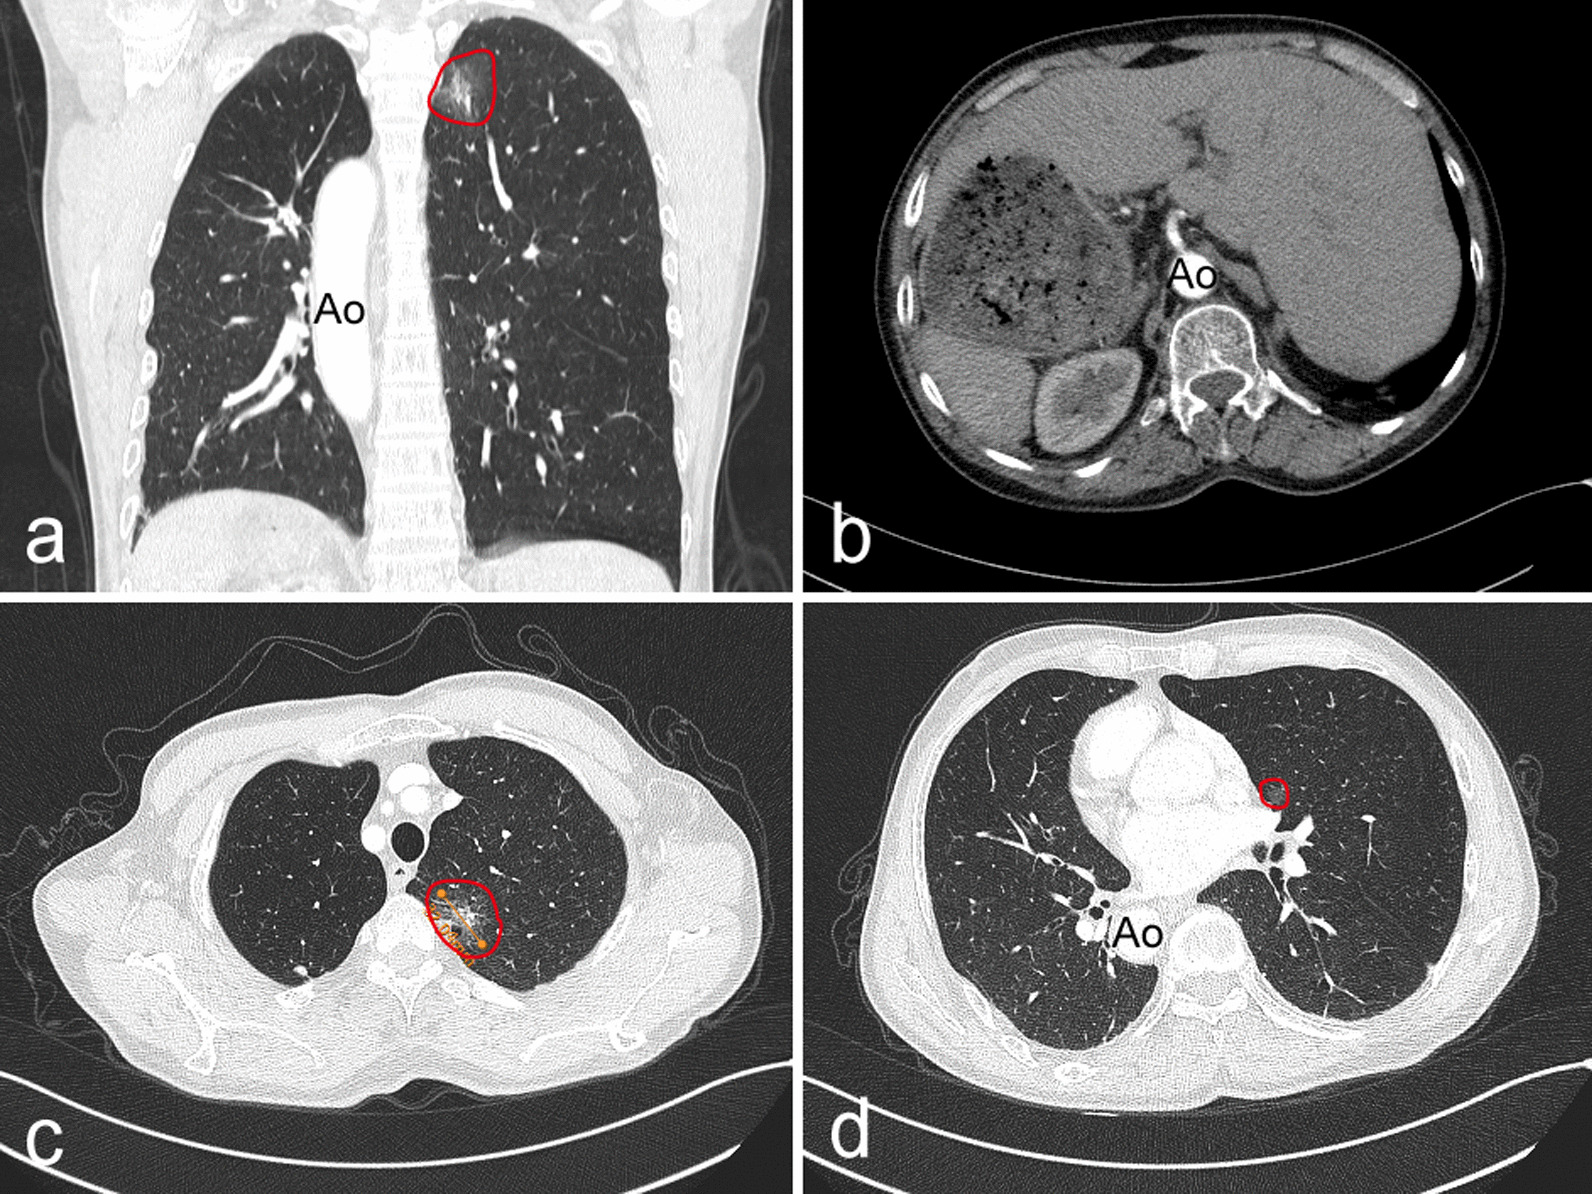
Computed tomography (CT) images. a: a mixed ground-glass opacity (mGGO) (measuring 34 × 32 mm in diameter) in the left upper lobe and mirror image of the aotic artery in the coronal section. b: abdominal organs presented with complete situs inversus. c: mGGO in the left upper lobe in the sagittal section. d: a pure ground-glass opacity (pGGO) measuring 0.8 cm in diameter closer to the mediastinum in the medial segment of left middle lobe
